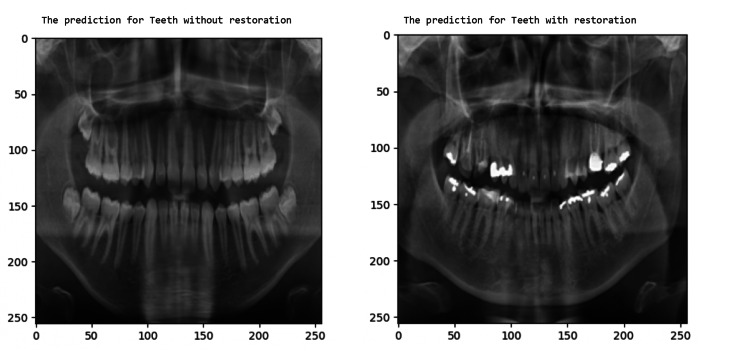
Dental radiographs with restoration predicted by the model
